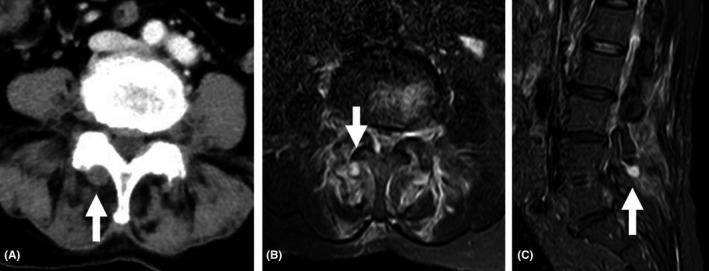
(A) Enhanced computed tomography shows a low‐density area in the right L4‐L5 facet joint (arrow). (B,C) MRI images show a high‐intensity area in the same region
